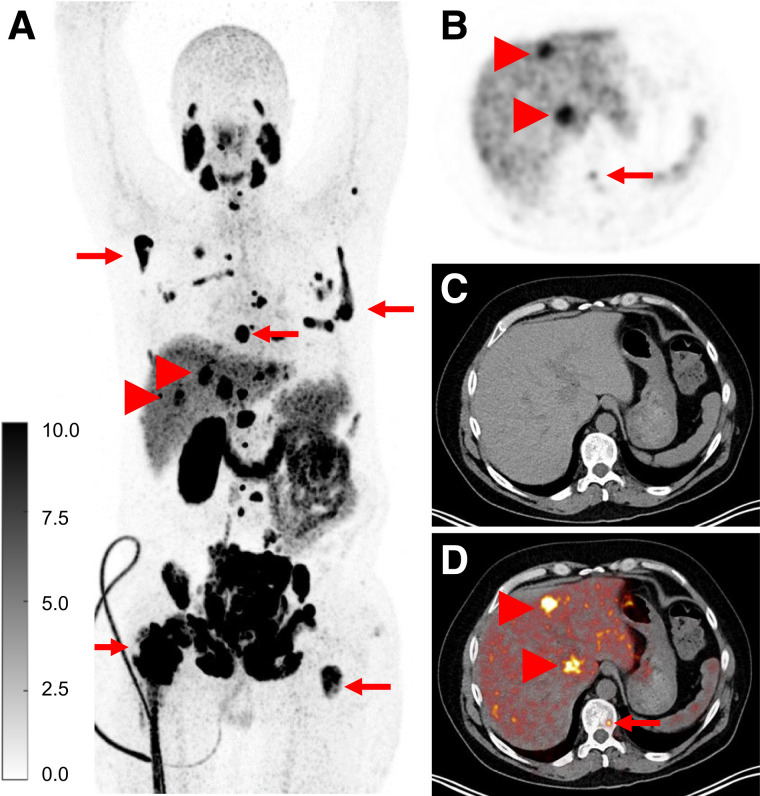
Administration of [^61^Cu]Cu-NODAGA-PSMA-I&T, in 48-y-old man with metastatic castration-resistant prostate cancer with disease progression, after abiraterone and docetaxel therapy before ^177^Lu-labeled PSMA radiopharmaceutical therapy. Imaging was performed 3 h after tracer administration. (A–D) Maximum intensity projection (A), and PET (B), CT (C), and fused PET/CT (D) images demonstrate multifocal osseous metastases (arrows) and hepatic metastases (arrowheads). Patient has also 1 kidney after left nephrectomy.
